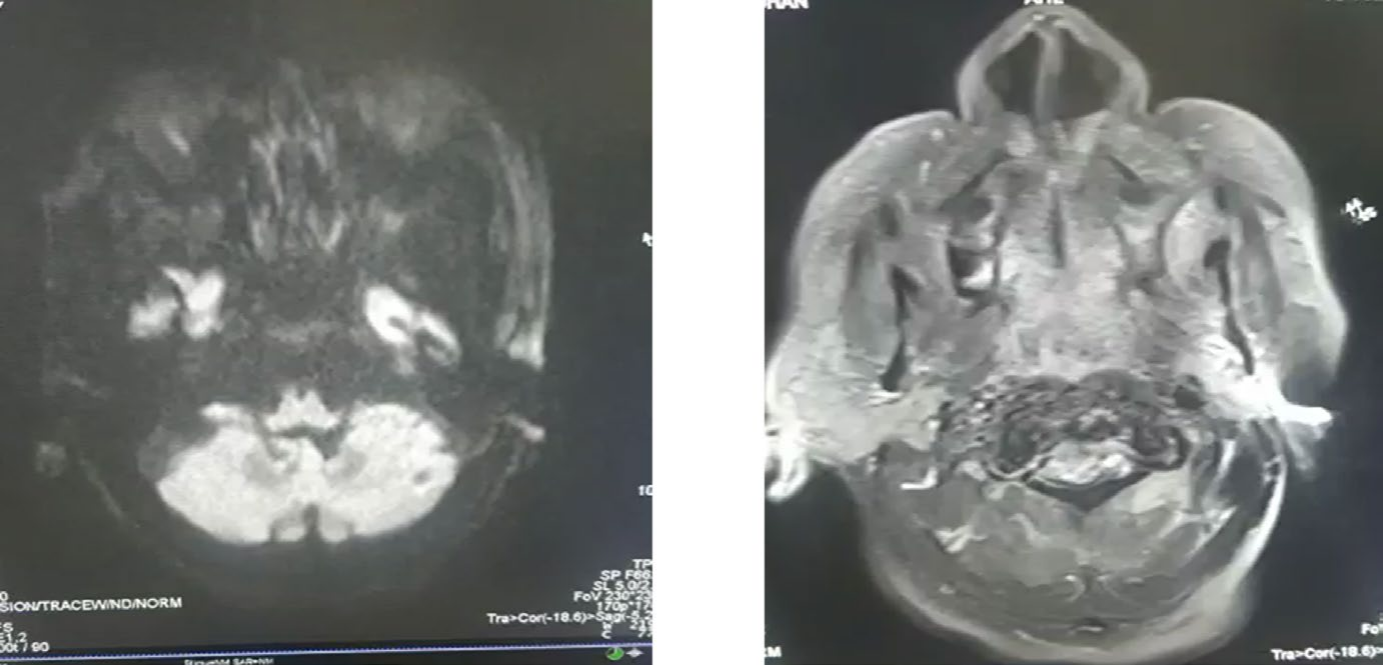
MRI of the brain showed signal alterations with restricted diffusion in the bilateral cerebral cortex, basal ganglia, and caudate nucleus, indicative of ischemia and cortical necrosis, likely resulting from hypoxic–ischemic injury.
